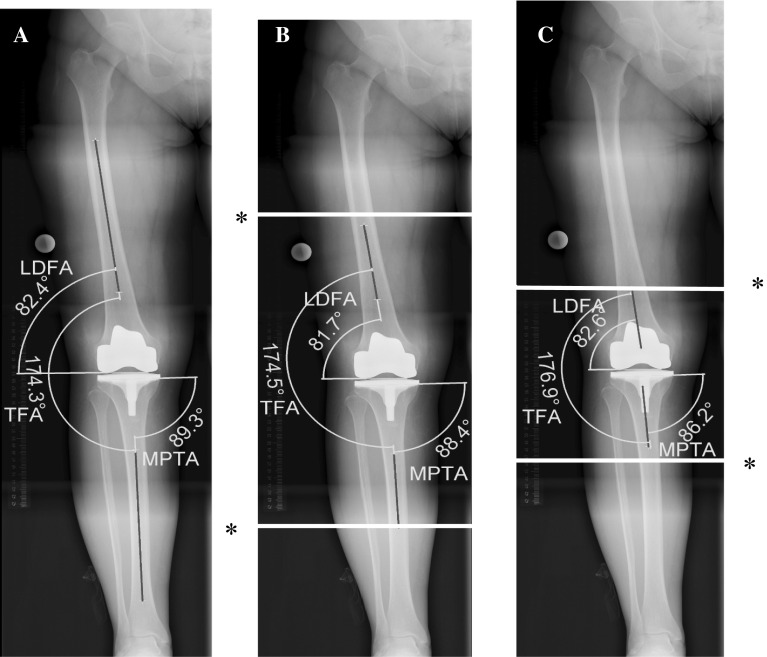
Measurement of TFA, MPTA and LDFA on a HTA radiograph, b 20 cm bone length radiograph, c 10 cm bone length radiograph. *TFA* tibial femoral angle, *LDFA* lateral distal femur angle, *MPTA* medial proximal tibial angle, *HTA* hip-to-ankle radiograph
